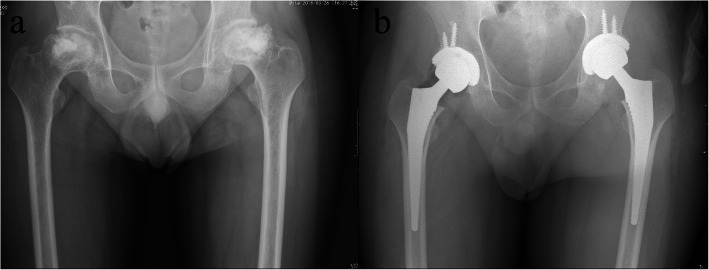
The anteroposterior radiographs of bilateral hip joints before (a) and after (b) bilateral total hip arthroplasties. a Radiograph taken 2 days before THA showed bilateral femoral head collapse and secondary osteoarthritis, which indicated ARCO IV stage osteonecrosis of the femoral head; (b) radiograph taken immediately after bilateral THA showed satisfactory location and fixation of bilateral femoral and acetabular prostheses
